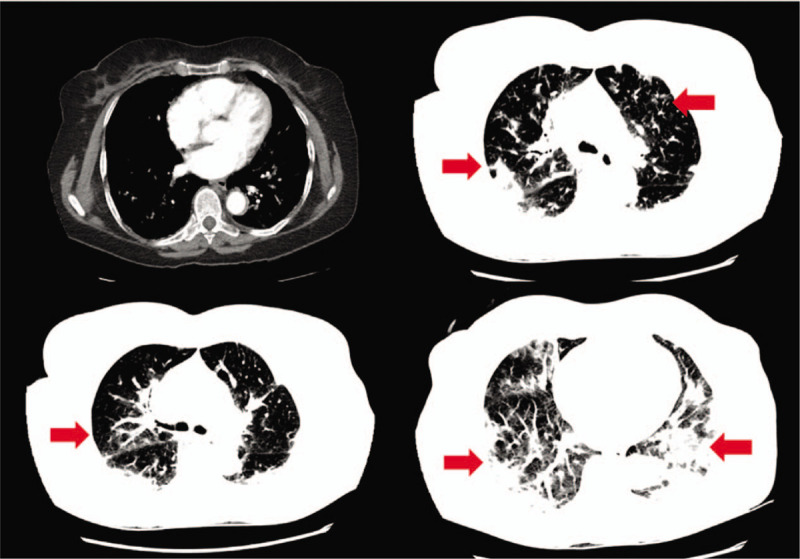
CT scan of the chest showing bilateral ground glass opacities, worsening in the lower lung fields.
